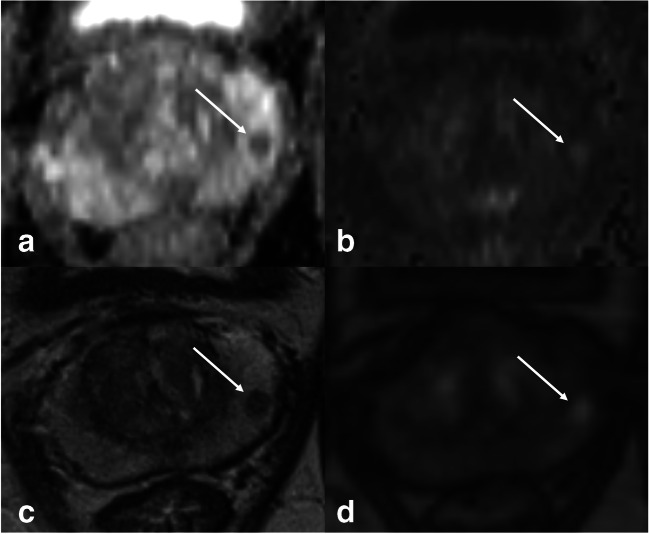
71-year-old man with a biopsy-proven ISUP 2 invasive prostate cancer visible on multiparametric MRI (white arrows). The lesion measures < 15 mm and was classified as PI-RADS 4 with good visibility on all sequences
